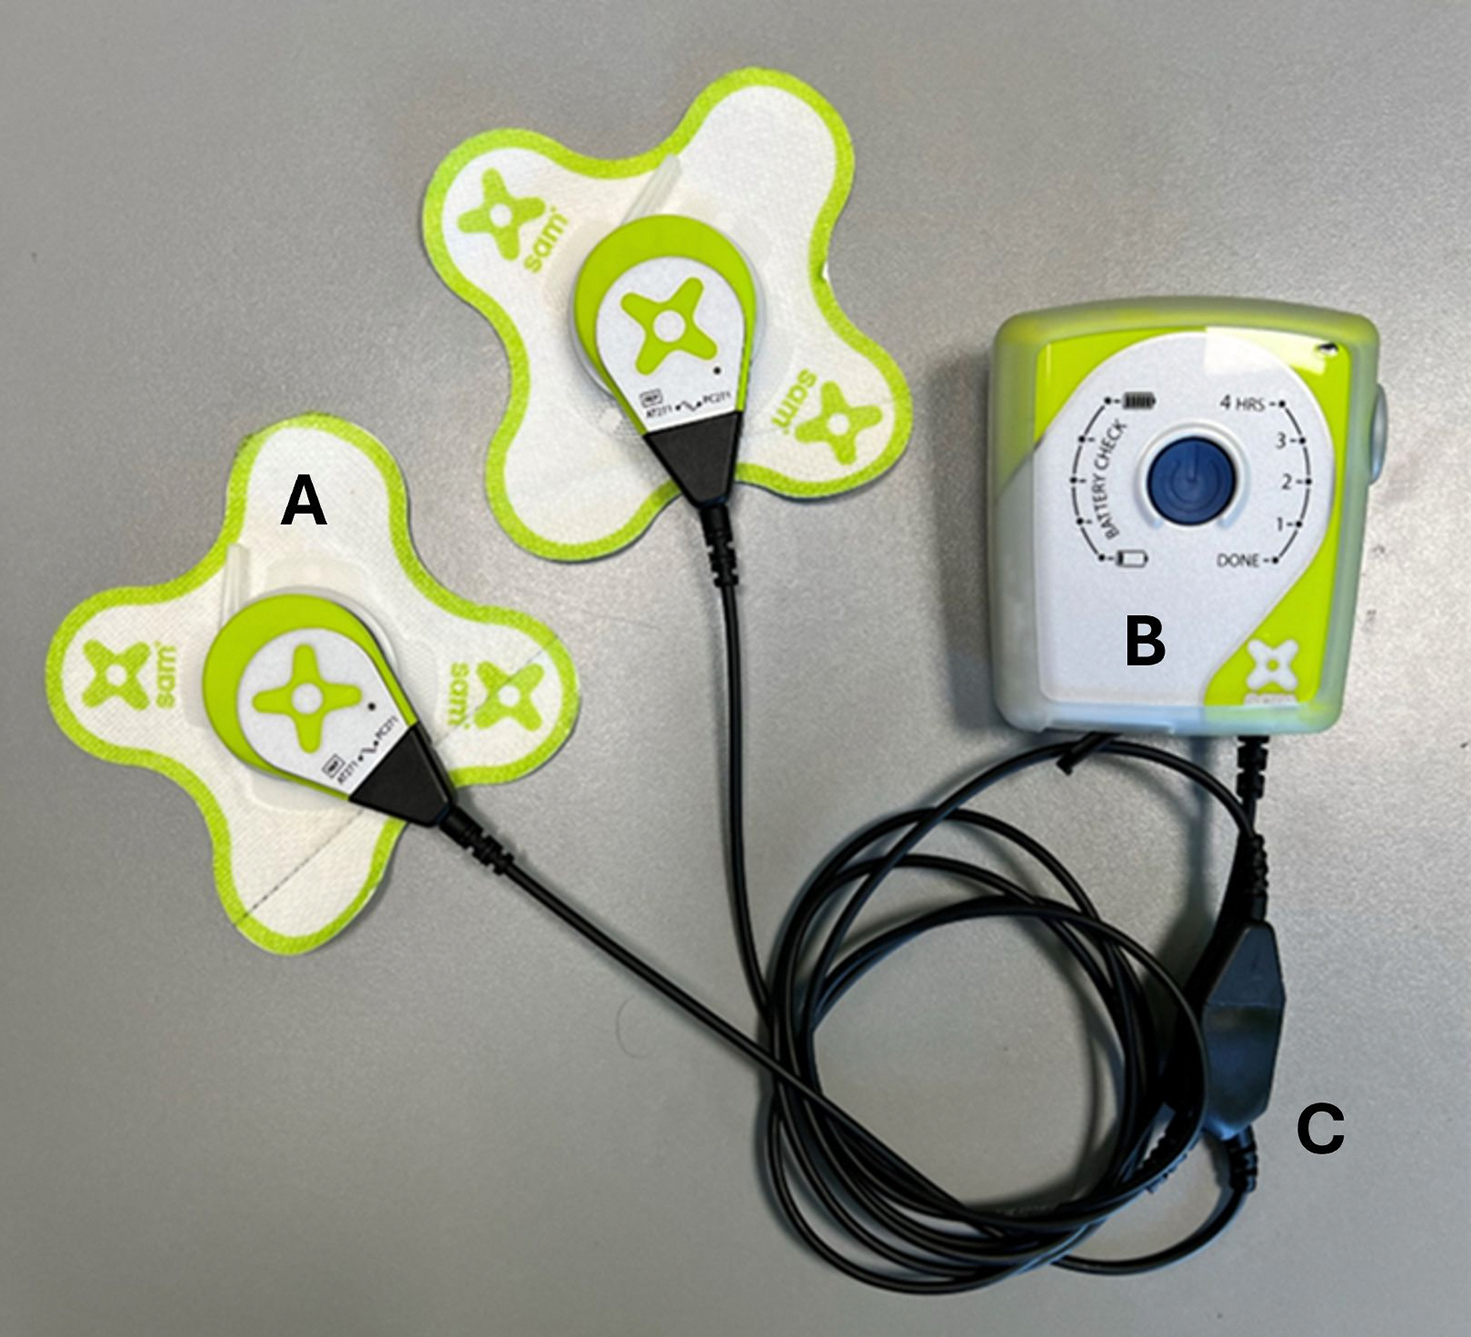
Basic 3 MHz LDU delivery system components for treating soft tissue injuries. A) Ultrasound transducer applicator and coupling patch, B) Ultrasound power controller and treatment timer, and C) Power splitter cable.
